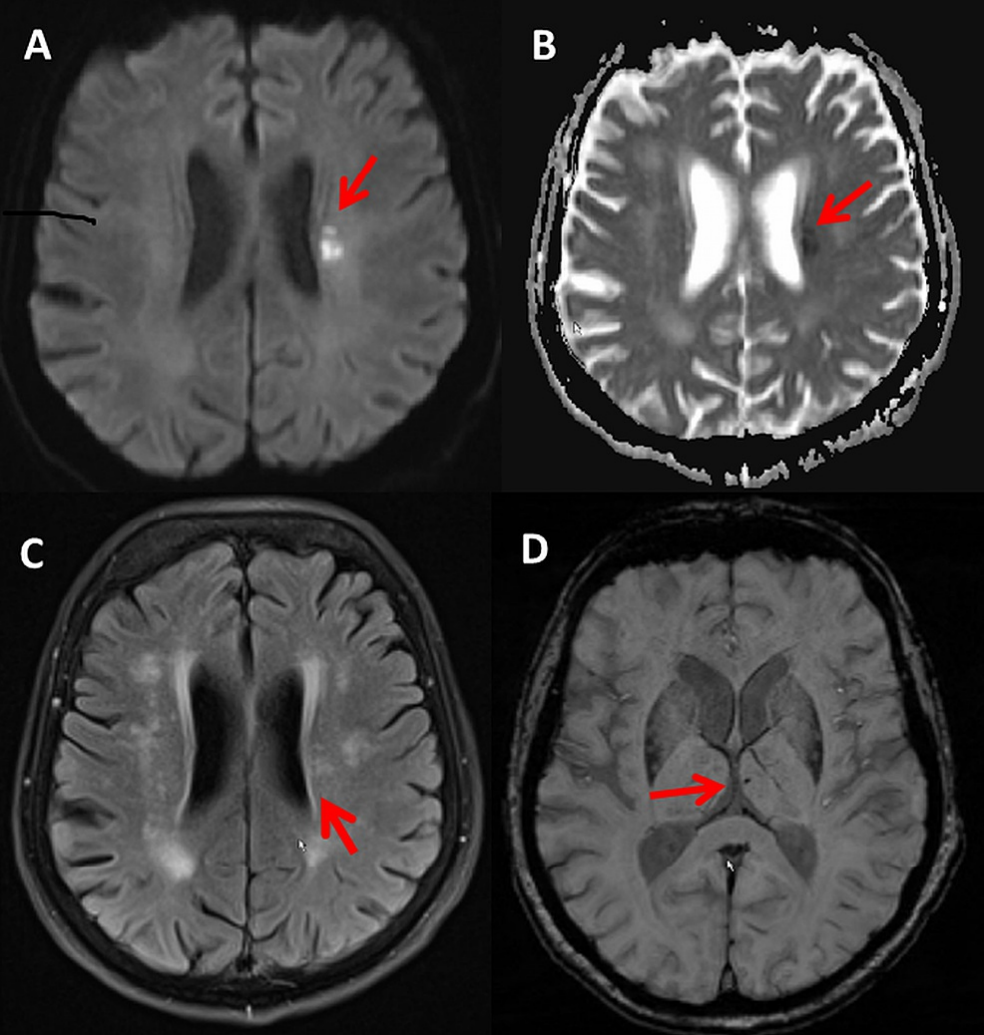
Cerebral MRI showing an acute stroke in the left periventricular region and a microbleed in the left thalamus (A: DWI, B: ADC, C: T2-weighted, D: SWI) DWI: diffusion-weighted imaging; ADC: apparent diffusion coefficient; SWI: susceptibility-weighted imaging
